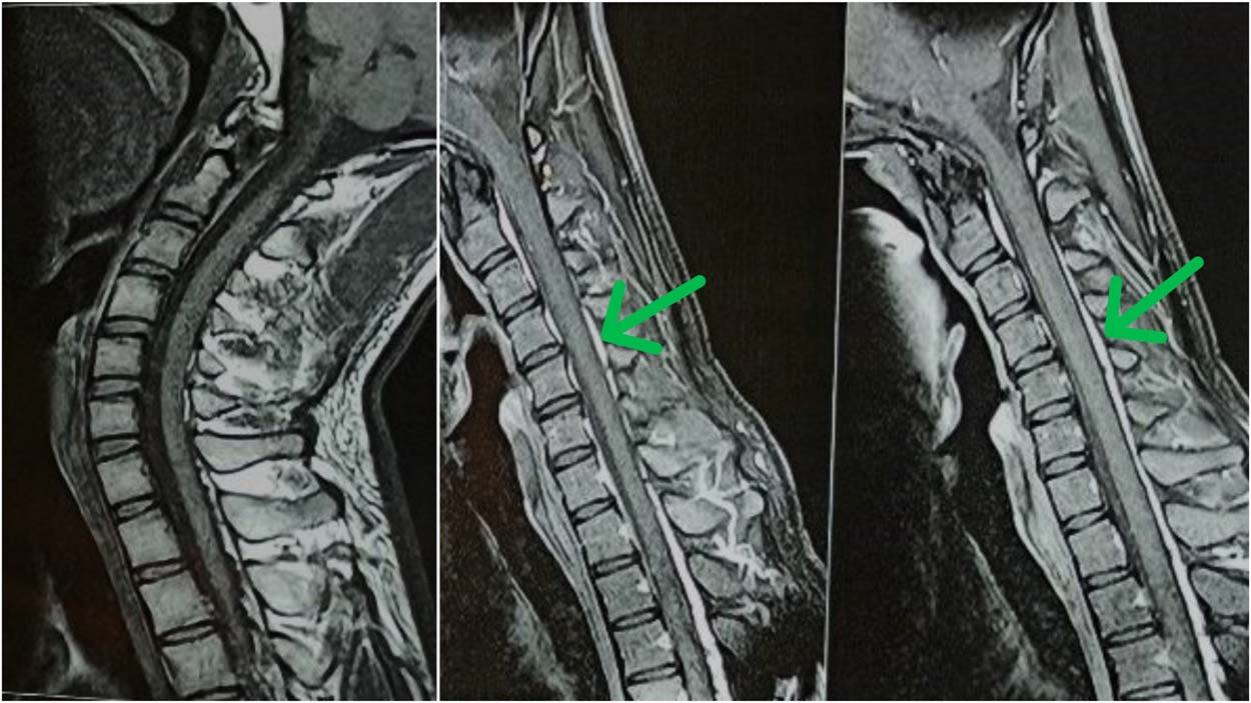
MRI of C-Spine (Left: Extension; Middle and Right: Flexion) shows anterior displacement of the dura with prominent epidural space from C4 to C6 level in flexion sequence with enhancement on post contrast study, no signal changes in spinal cord.
